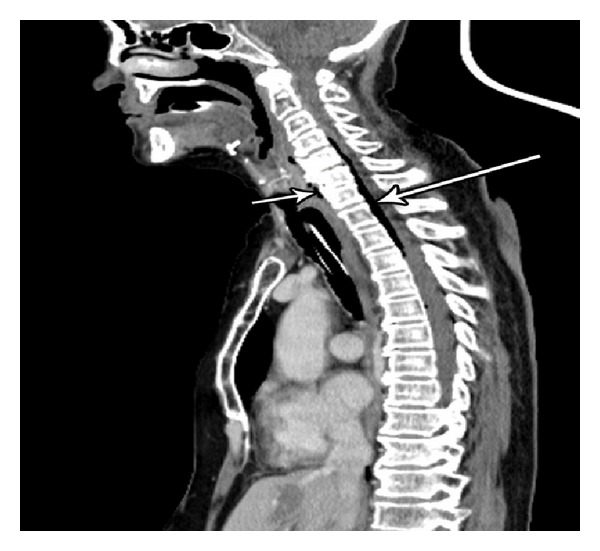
Postcontrast sagittal CT demonstrating air in the prevertebral space (short arrow) and spinal canal (long arrow).
